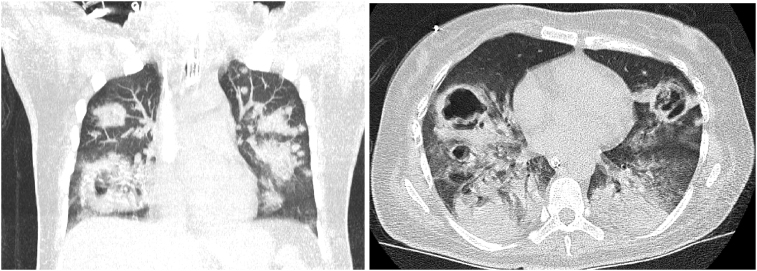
CT Chest on HD11. Left: coronal. Right: axial. Showing new large areas of cavitation bilaterally with some surrounding groundglass halos, consistent with necrotizing pneumonia, possibly invasive aspergillus.
